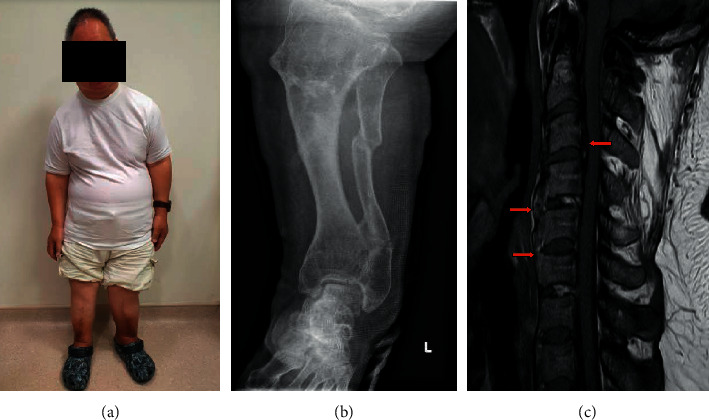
(a) Short stature, (b) deformities of the lower limb with recent fibular fracture, and (c) MRI cervical spine showing ossification of the anterior longitudinal ligament from C3 to C6 and posterior longitudinal ligaments from C2 to C4 (arrows).
